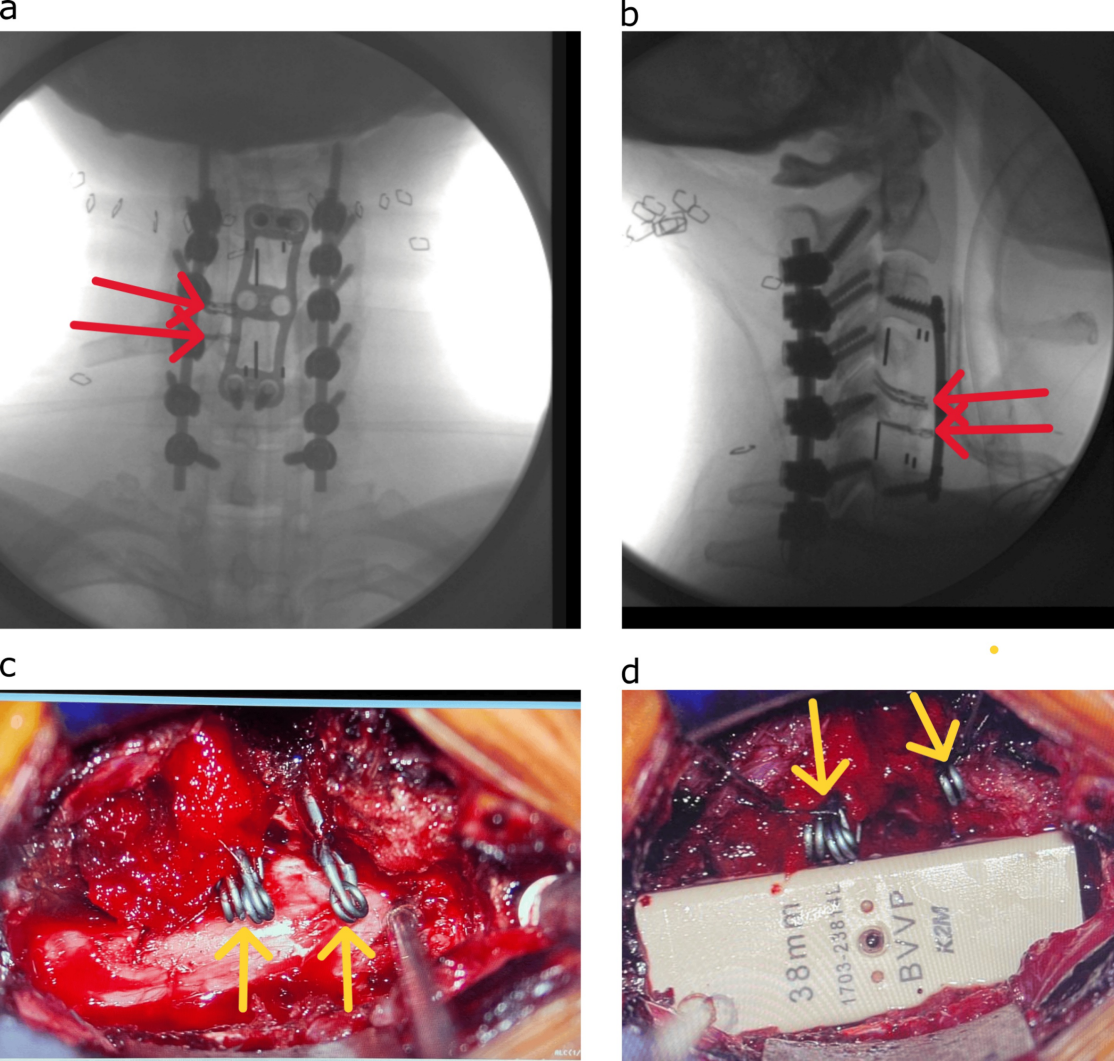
Intra-operative images (a) Intra-operative X-ray (anterior-posterior view) demonstrating the anterior-posterior and lateral images of the operative construct. Note the presence of the three aneurysm clips (red arrows). (b) Intra-operative X-ray (lateral view) demonstrating the anterior-posterior and lateral images of the operative construct. (c) The aneurysm clips underlying the surgically modified interbody cage (yellow arrows). (d) Interbody cage. Note the presence of the three aneurysm clips (yellow arrows).
